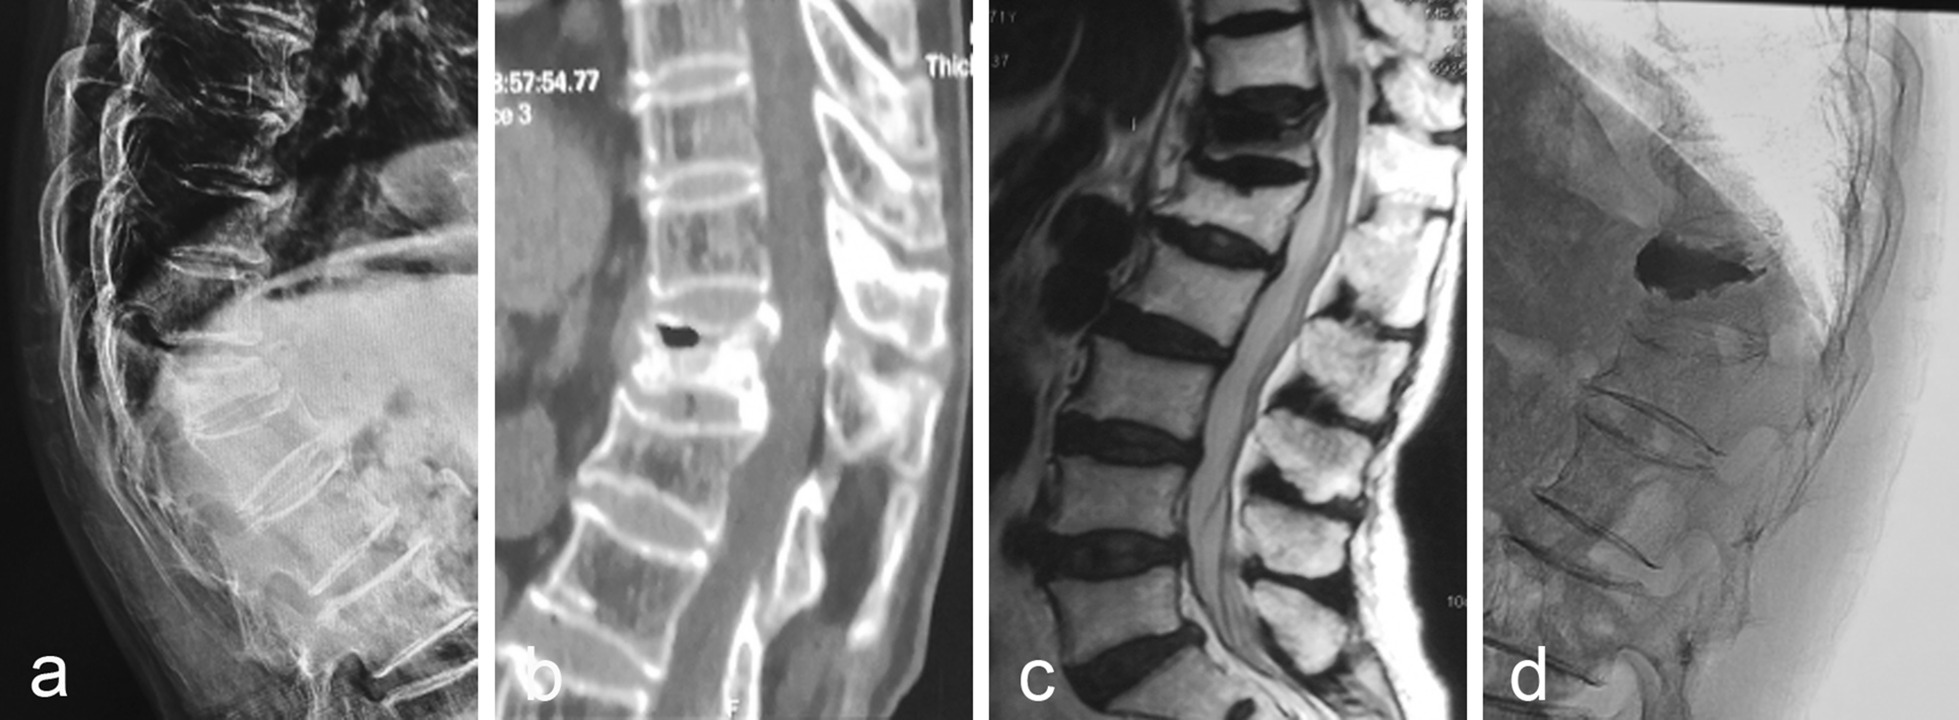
A 71-year-old female patient (Grade I) presented with minor trauma 5 months ago and low back pain 2 months ago, which was associated with activity. a The X-ray showed wedge-shaped changes of the T12 vertebra with kyphosis of the spine; b CT showed anterior collapse of the T12 vertebra with low-density shadow and peripheral sclerosis; c MRI T2 showed low signal in the vertebral body; d Postoperative X-ray showed that the height of the injured vertebra recovered well and the kyphosis deformity was corrected
